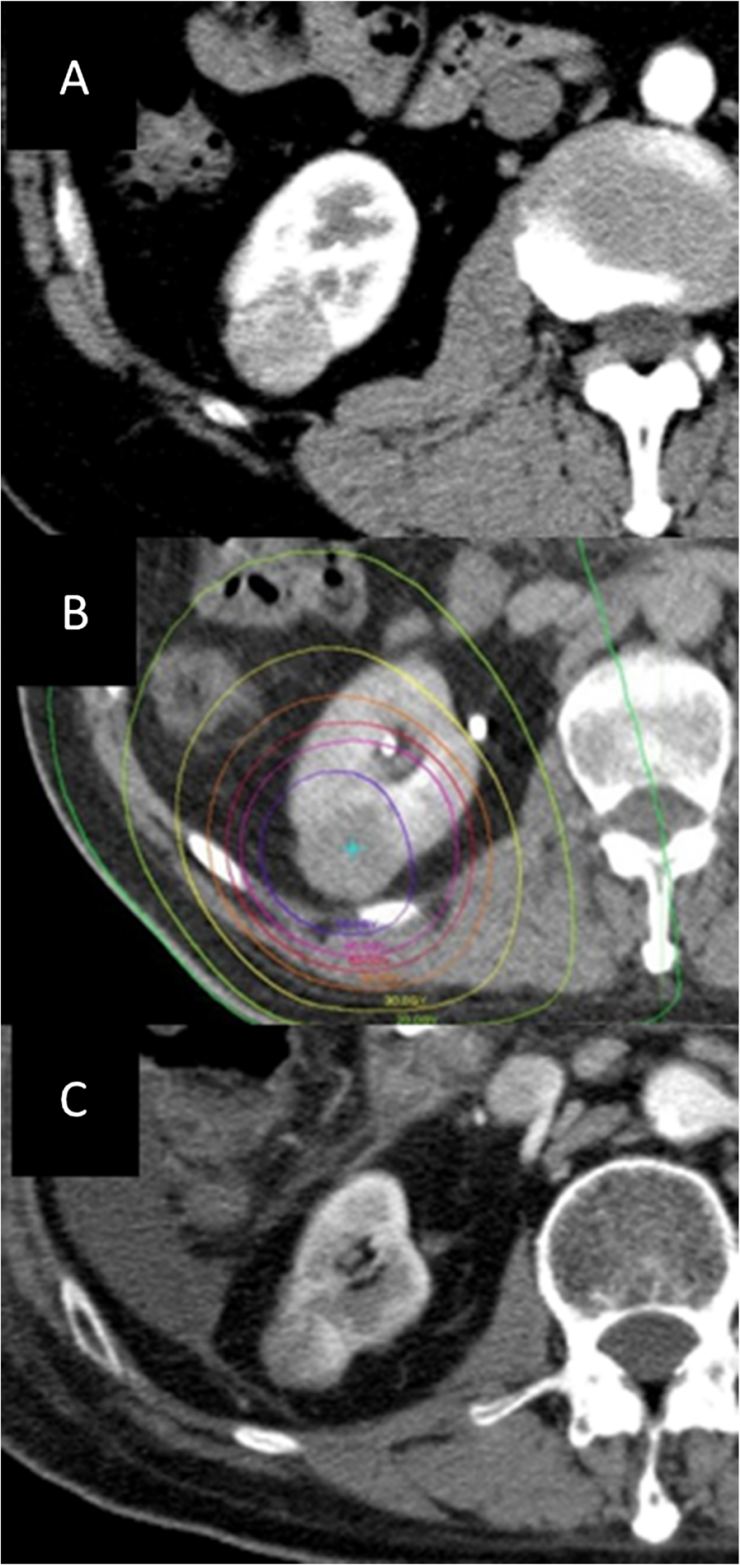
Initial CT, radiotherapy planning, and follow-up CT. (A) Axial contrast-enhanced computed tomography image before stereotactic body radiation therapy. (B) Dose distribution of the planning for stereotactic body radiation therapy. Isodose lines show 70Gy, 60Gy, 50Gy, 40Gy, 30Gy, 20Gy, and 10Gy from the inside. (C) Axial contrast-enhanced computed tomography image two years after SBRT. The renal tumor decreased in size and its surrounding renal portion got atrophic.
